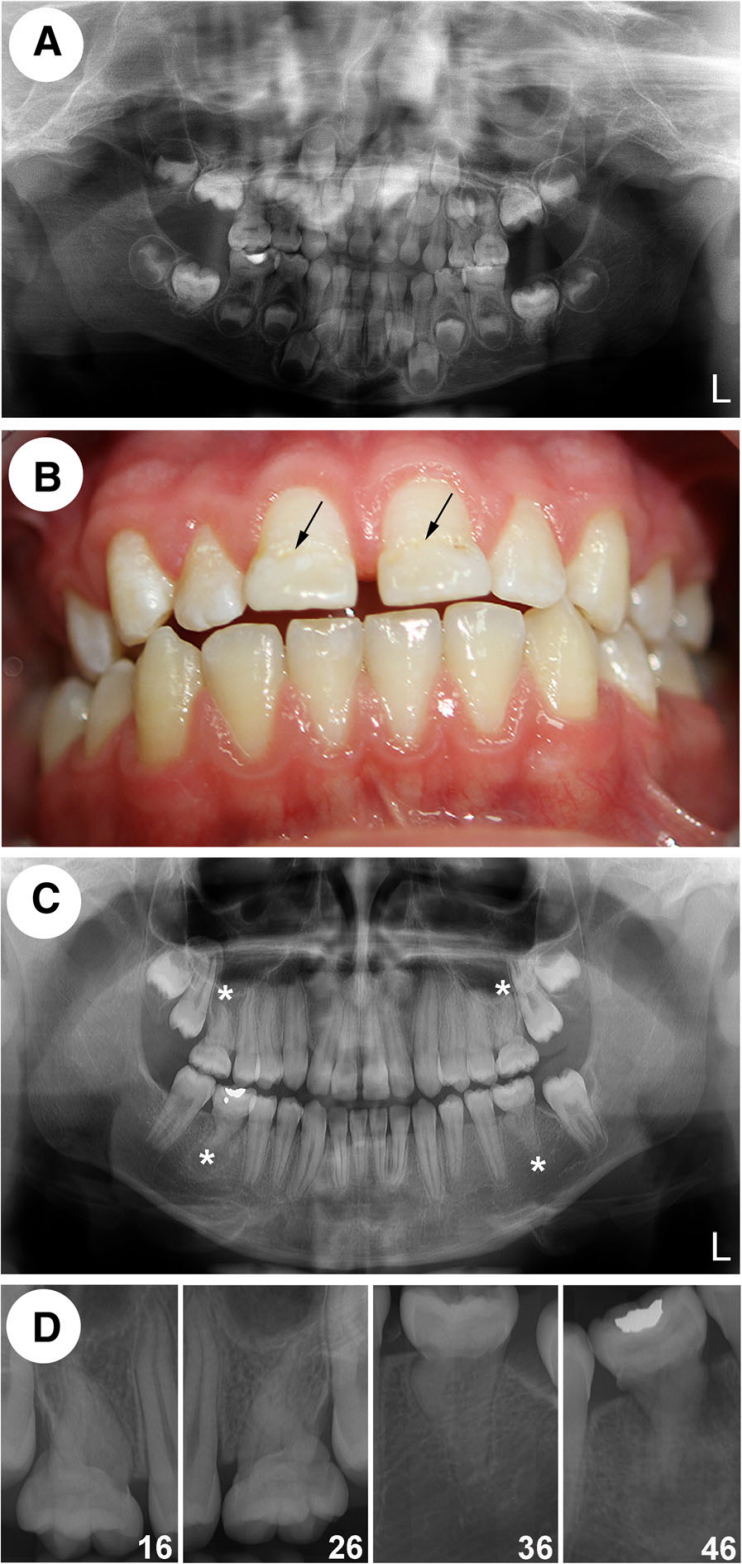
Radiographic and clinical characteristics of MRIM in a patient with ALPS. (a) A dental panoramic tomogram (DPT) taken when the patient was 6 years old reveals small pulp chambers and thin, short roots of all four primary second molars. In all four second primary molars, only thin horizontal lines can be seen presenting dental pulp chambers. The lack of dental pulp is more pronounced in both lower second primary molars. Comparison of the volumes of the dental pulps between the first and second primary molars reveals a substantial difference, with the second primary molars having a much smaller dental pulp chamber. Fillings are apparent on the primary mandibular second molars. Under the filling on the right side, there is a secondary caries lesion with no signs of periapical inflammation. (b) An intraoral image of the patient at the age of 12 years shows the permanent dentition. The tooth crowns show normal morphology except for the hypoplastic incisal thirds of the upper central incisors, which were both built up shortly upon eruption (marked with arrows). (c) A DPT taken at the same age (12 years) shows that in all four permanent first molars (PFMs), the pulp chambers are hardly recognizable and appear to be constricted into a narrow straight form and that the roots of these teeth are shorter and thinner than normal. (d) Periapical radiographs reveal barely visible contours of the dental pulp of all four PFMs. In particular, the lower PFMs show severely aberrant roots with no identifiable pulp chambers. In tooth 46, periodontal inflammation is visible, most likely a sequel of the aberrant tooth root
